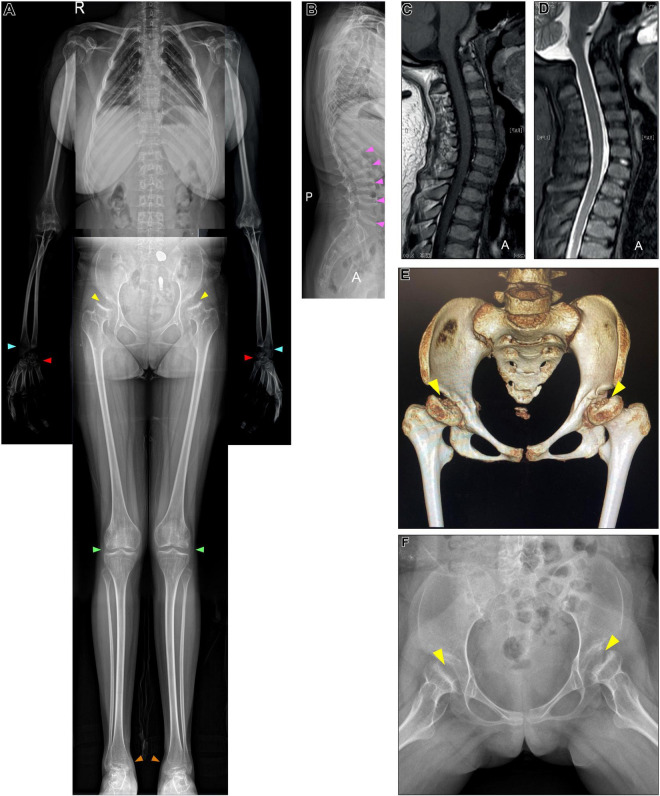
X-ray, CT of the pelvis, and cervical MRI examination. (A,B) Whole-body X-ray found multiple skeletal deformities, including vertebral wedging (pink arrows), small irregular carpal bones (red arrows), the distal portion of the radius being tilted toward the ulna (blue arrows), mild genu valgum (green arrows), and ankle valgus (orange arrows). (A,F) Pelvis X-ray and (E) CT collectively show bilateral acetabular dysplasia, and femoral heads collapse (yellow arrows). (C,D) MRI excluded cervical stenosis.
